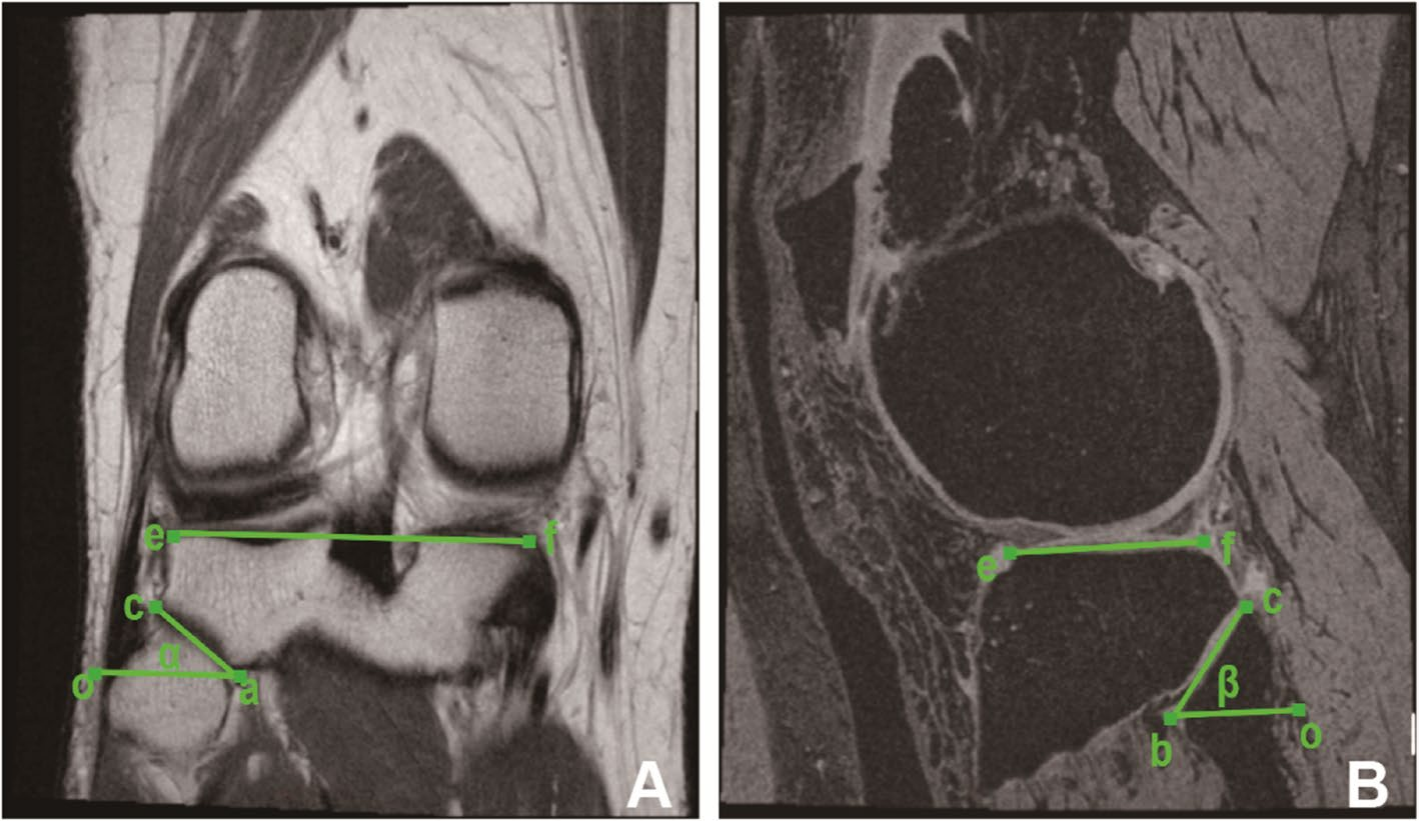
Measures of proximal tibiofibular joint (PTFJ) morphological parameters. A T1-weighted coronal MRI slices of the knee. Point *c* is the outermost point of the fibular articular surface, and *a* is the innermost point of the articular surface in PTFJ. *e* is the lateral vertex of the tibial plateau, and *f* is the medial vertex of the tibial plateau. Line *oa* is drawn through *a*, which is parallel to line *ef*. The length of *ac* and the angle *oac* (α) are measured in each slice of MRI. B Sagittal fat-suppressed T1-weighted 3D gradient-echo MRI. Point *b* is the lowest point of the fibular articular surface, and *c* is the highest point of the fibular articular surface in PTFJ. *e* and *f* are points that connect the anterior and posterior horns of the lateral meniscus with the tibial plateau, respectively, and line *ef* is parallel to the tibial plateau. Line *ob* is drawn through *b* and is parallel to *ef*. The length between *bc* and the angle of *obc* (β) are then measured in each slice of MRI
